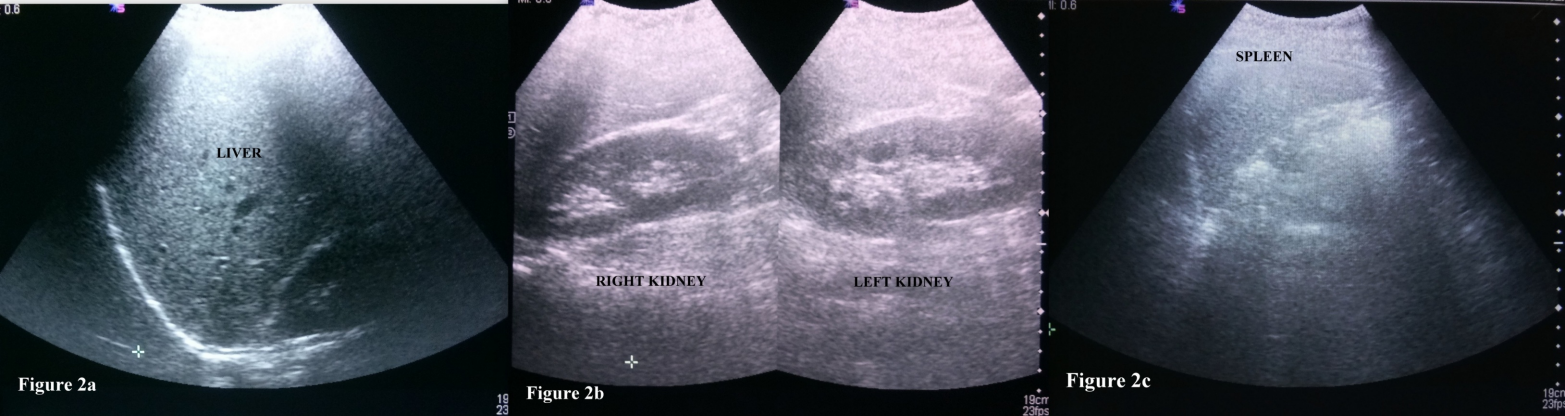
Ultrasound abdomen of the patient with belly dancer's dyskinesia Figure 2a showing normal liver size and echotexture. Figure 2b showing bilateral kidneys having normal size and preserved corticomedullary differentiation. Figure 2c showing spleen with normal size and shape
